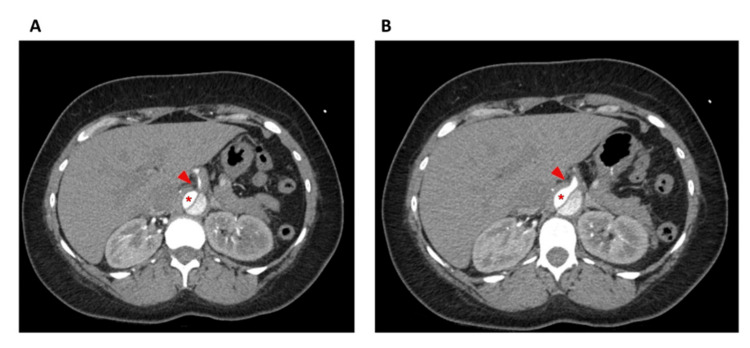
Computed tomography imaging demonstrated dissection notably extending into the celiac axis Red arrow shows celiac trunk; * indicates true lumen
